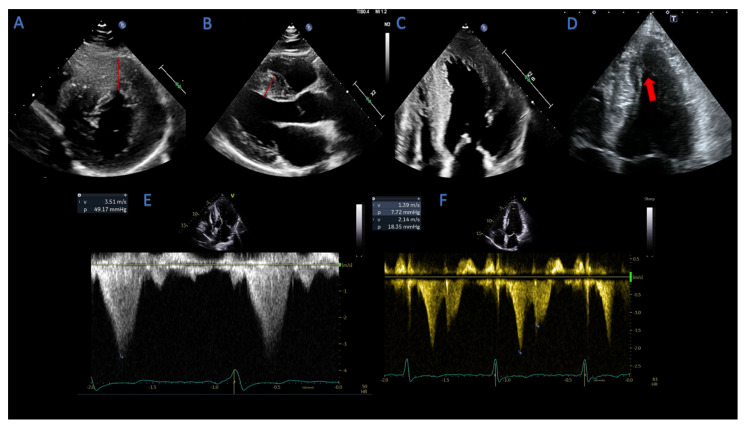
Echocardiographic patterns of hypertrophic cardiomyopathy. (A) focal asymmetric anterior wall hypertrophy (red line), parasternal short axis view; (B) focal asymmetric septal hypertrophy (red line), parasternal long axis view; (C) concentric hypertrophy pattern; (D) apical hypertrophy pattern (arrow); (E) dagger-shaped profile of CW doppler in LVOTO; (F) lobster claw profile of CW doppler in mid cavity obstruction.
